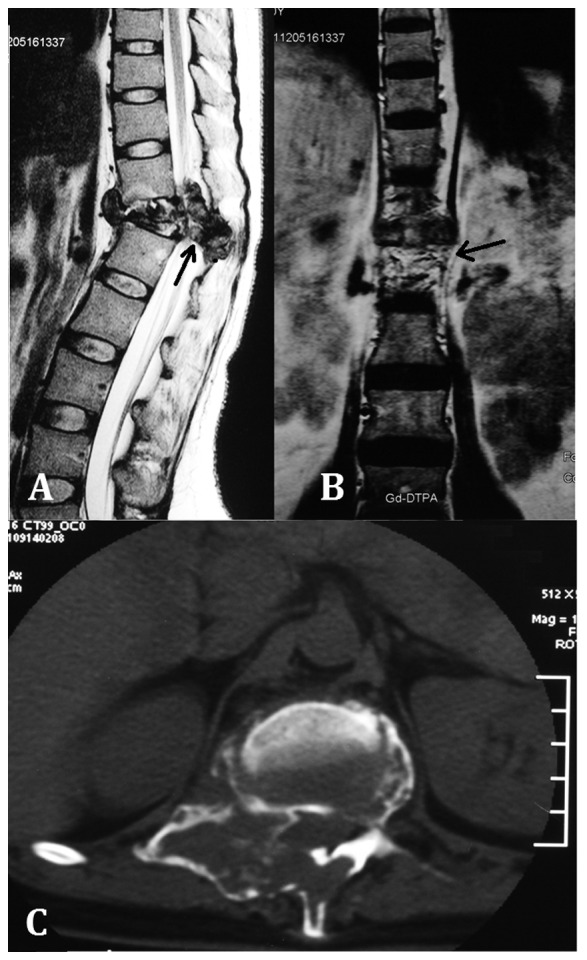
Case two. (A) Sagittal, (B) coronal and (C) transverse plane magnetic resonance imaging showing the additional mass in the spinal canal and posterior column of the T12 vertebra.
